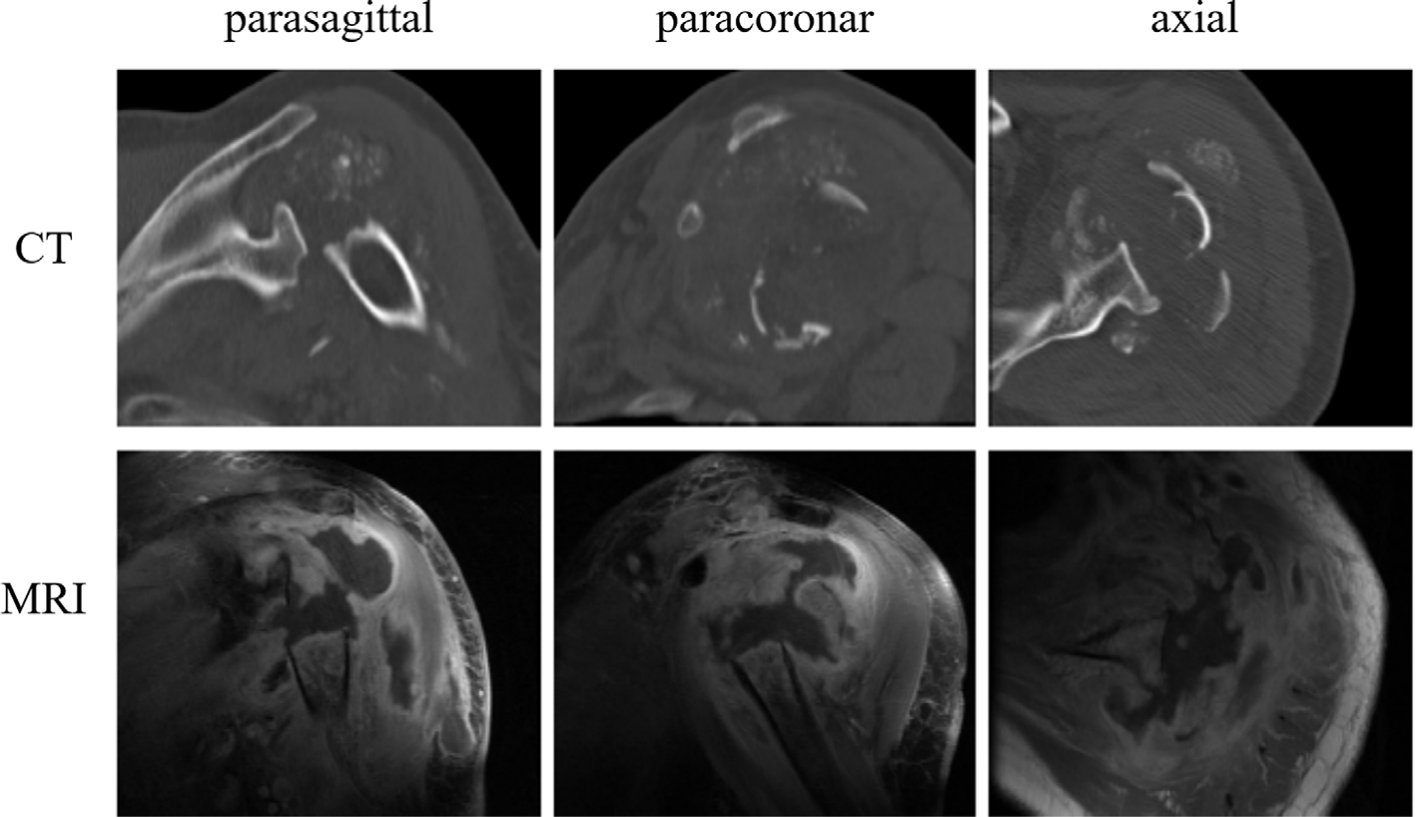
CT and MRI of the left shoulder (patient 1): massive osteolysis and tumour. Multiple similar calcifications were found in all three patients in CTs.
